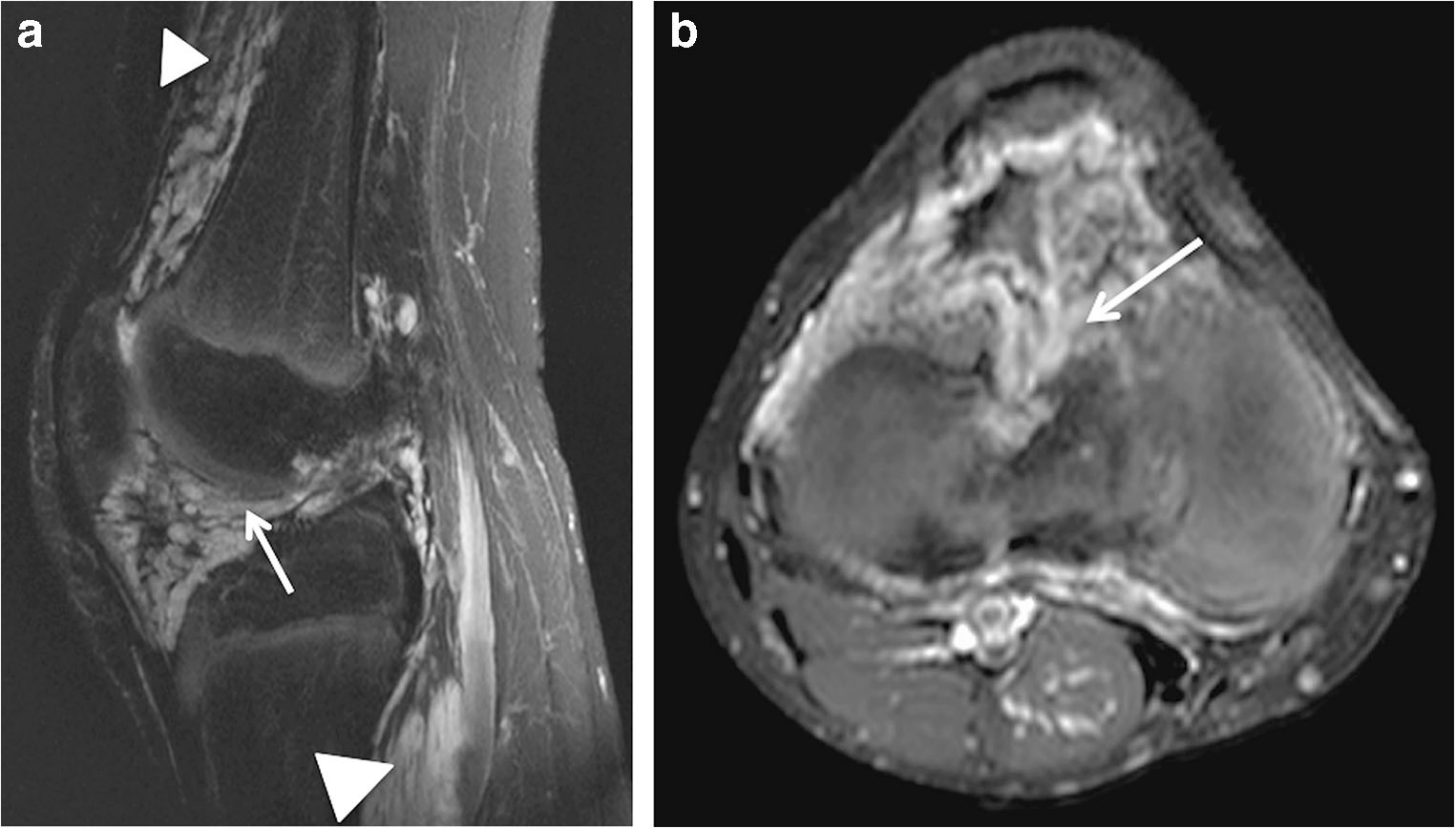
An 11-year-old boy with an extensive and diffuse venous malformation of the lower extremity. a A T2-weighted fat-saturated sagittal MRI demonstrates the involvement of all intracapsular spaces including the synovial cavity in the intercondylar space (*arrow*), as well as extracapsular muscle involvement (*arrowheads*). b A T1-weighted fat-saturated post-contrast axial MRI at the level of the intercondylar space demonstrates intensive enhancement of the ectatic veins of the malformation (*arrow*)
